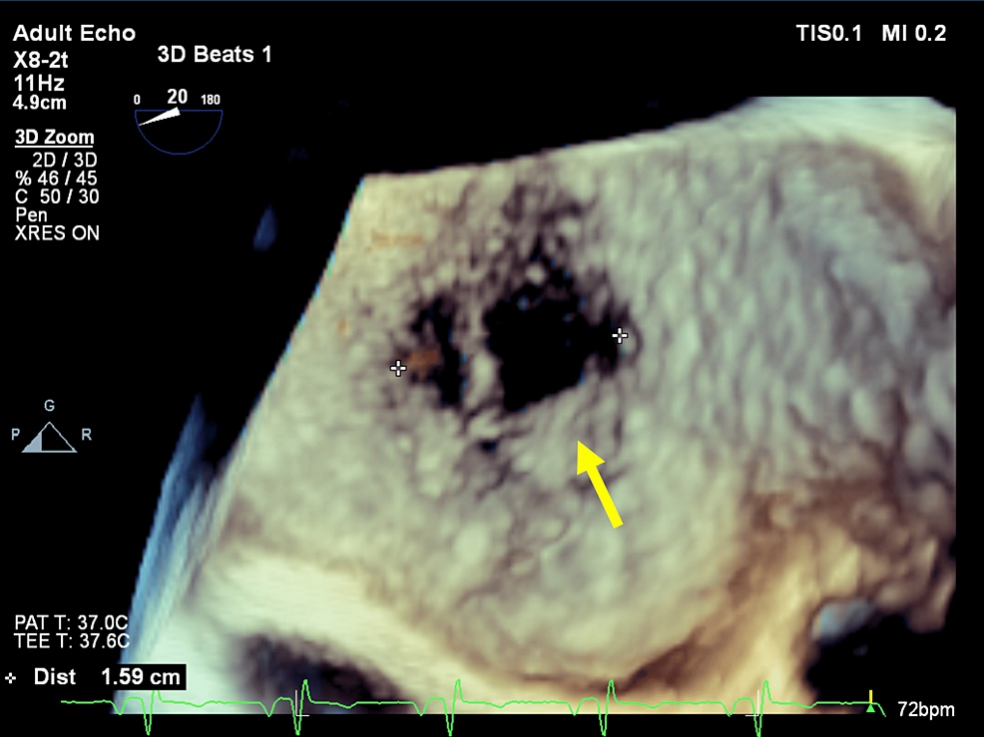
Three-dimensional TEE, mid-esophageal level, and four-chamber view (20o) demonstrate the large fenestrated ASD secundum type. TTE: Transthoracic echocardiography; ASD: Atrial septal defect.
